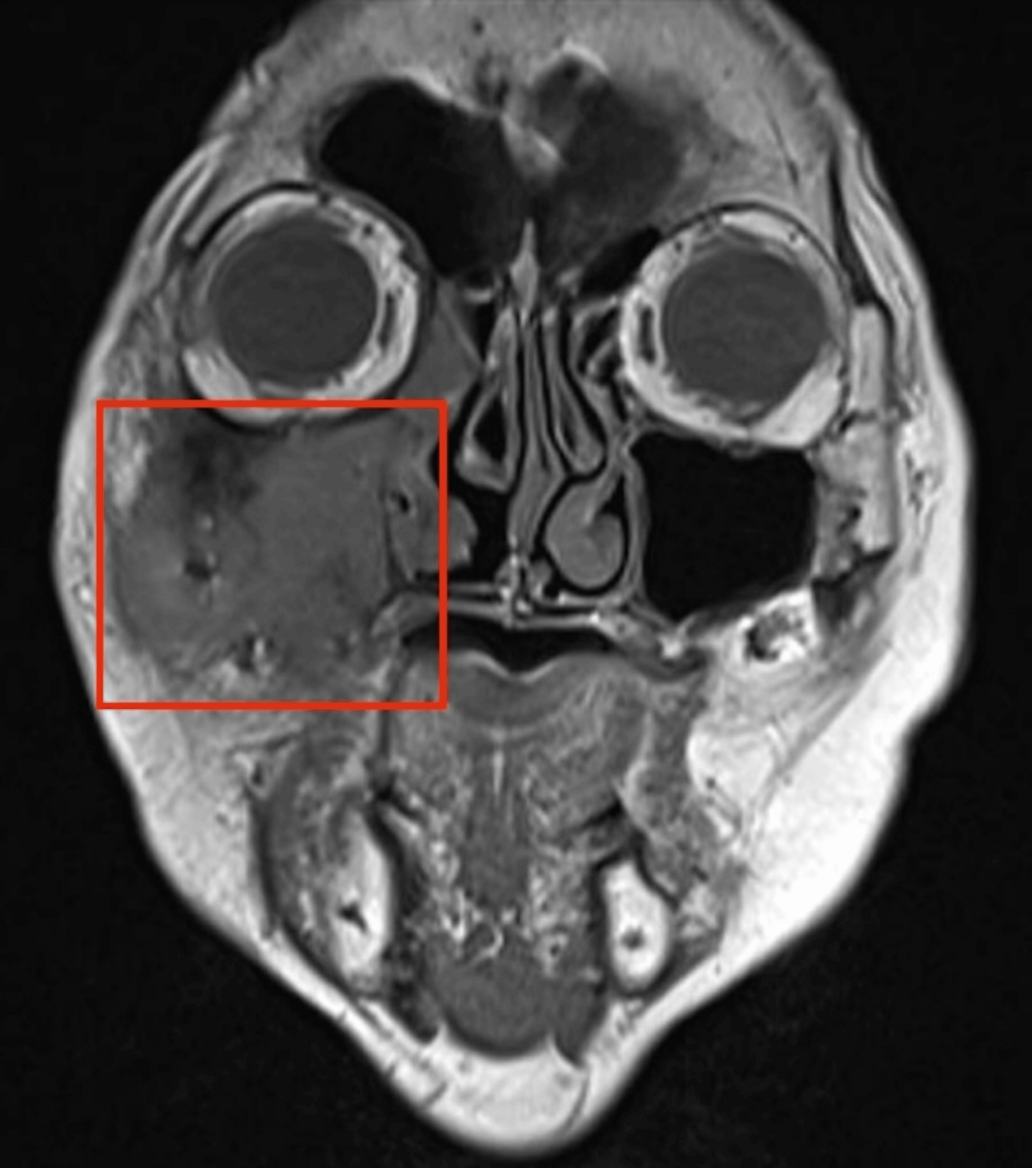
T1-weighted coronal MRI depicting a lobulated hypointense mass in the right maxillary sinus causing the destruction of the anterior and lateral maxillary walls, with extension into the pre-antral fat and buccal and masticator spaces MRI: magnetic resonance imaging
